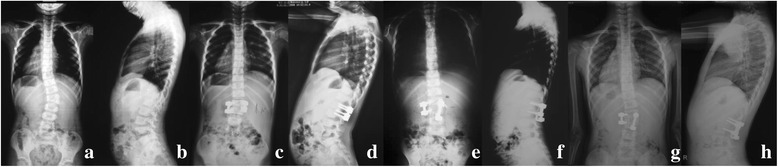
A 5-year-old girl with congenital scoliosis. Pre-operative radiographs (a, b) showed semi-segmented hemivertebra of L2. She complained of right leg pain on the third post-operational day. Post-operational radiographs (c, d) showed right L3 pedicle screw in wrong place. Revision surgery was performed with hooks, and his leg pain disappeared after the revision surgery (e, f). And it maintained well in 7 years follow-up (g, h)
